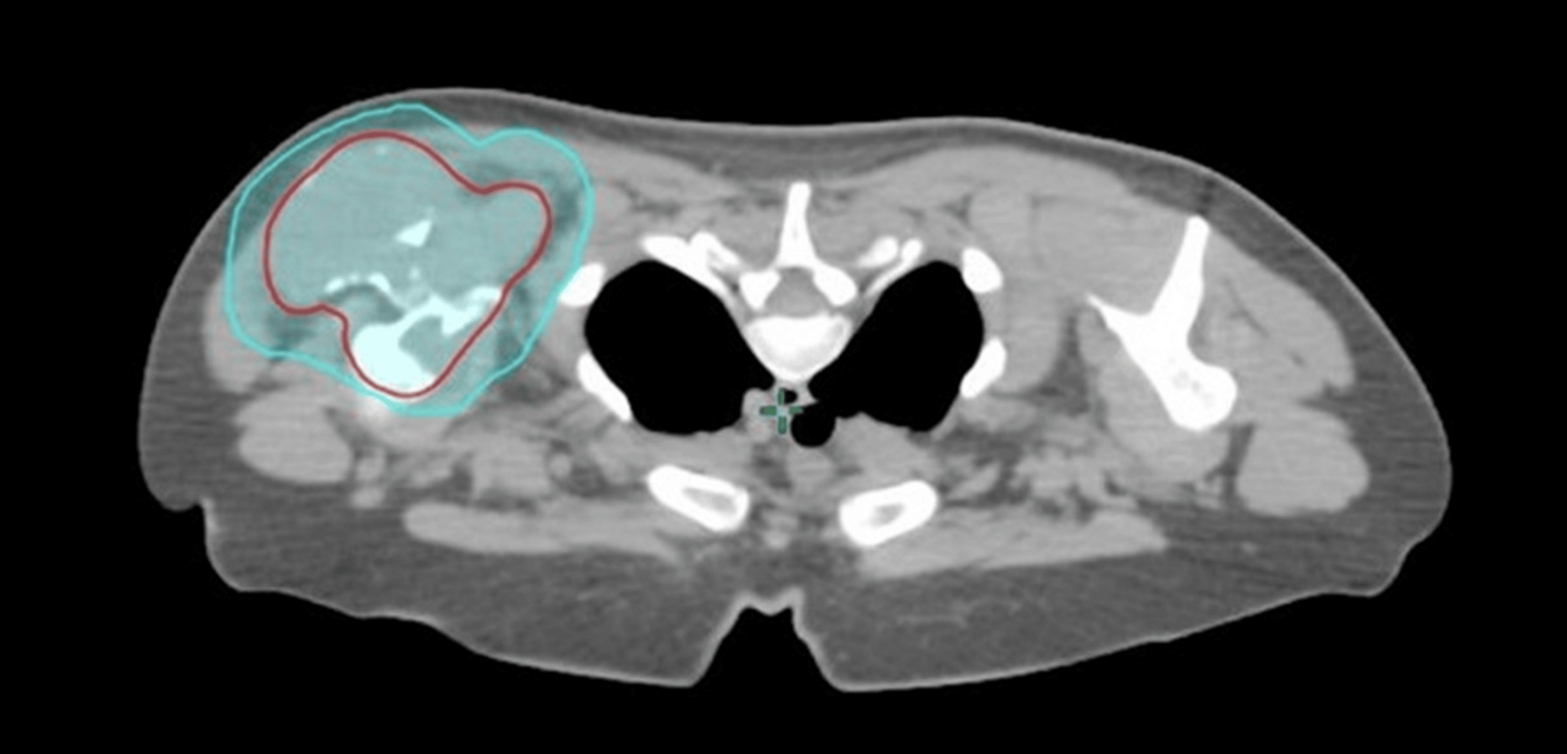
Axial CT image from the patient’s dosimetric scan showing target volume delineation with the CTV in red and the PTV in blue for the left shoulder plasmacytoma. CTV: Clinical Target Volume, GTV: Gross Tumor Volume
